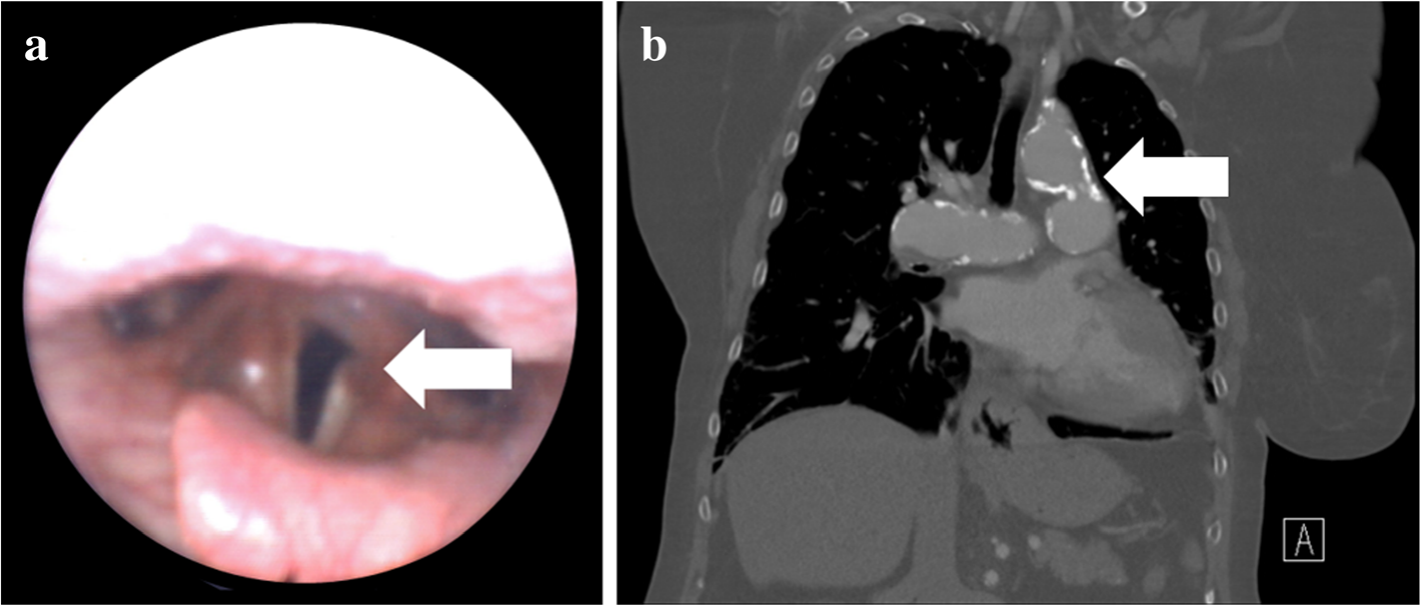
Physical examination and investigations, a) Visualization of the vocal cords by a flexible nasopharyngeal scope. Left immobile vocal cord seen in paramedian location; b) Coronal CT image showing a large partially calcified PDA with enlarged central pulmonary arteries responsible for putative mass effect and the Eisenmenger pathologies
